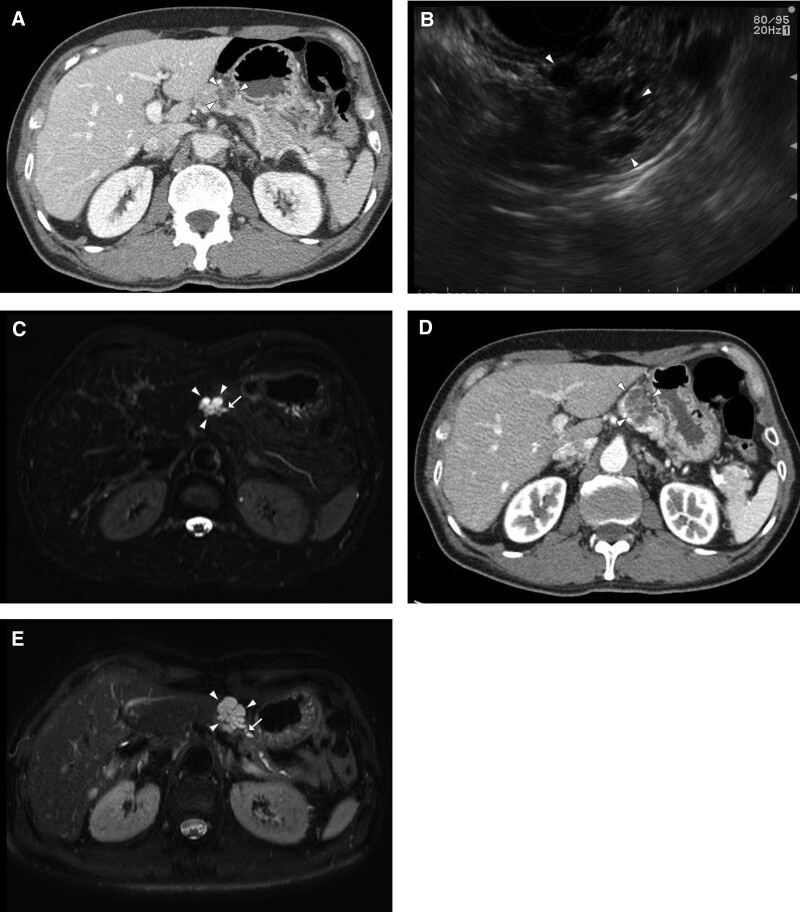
(A) Initial abdominopelvic computed tomography (APCT) revealed a roughly 2.2 cm sized irregular multilobulated low-density lesion (arrowheads) in the pancreatic body. (B) Endoscopic ultrasonography showed macrocystic (arrowheads), and honeycomb pattern with central sunburst-like appearance. (C) Pancreas magnetic resonance imaging (MRI) revealed that the main pancreatic duct (MPD) (D, arrow) appeared to be communicated with multilobulated cystic lesion (arrowheads) in pancreas. (D) Follow-up APCT showed that the pancreatic cystic lesion (arrowheads) gradually increased in size by 1.4 cm in the 4 years. (E) Additional pancreas MRI revealed that dilatation of the distal MPD (arrow) and the connection of MPD with the pancreatic cystic lesion (arrowheads) were more pronounced.
